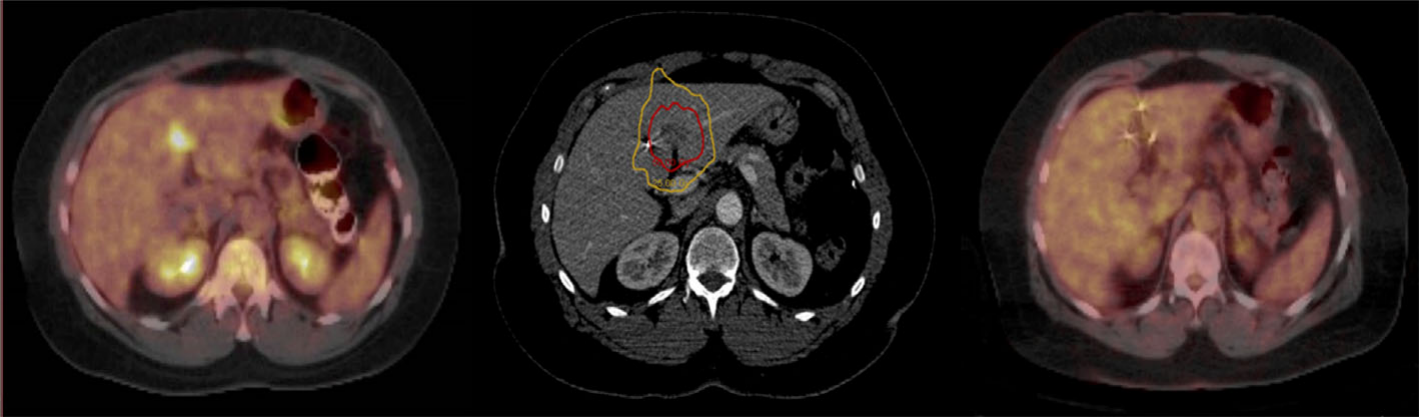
Pre-treatment PET/CT with a single liver metastasis (Left); Red prescription isodose line encompassing the liver metastasis (Center); PET/CT at 6 months post-treatment demonstrating eradication of the liver metastasis (Right).
